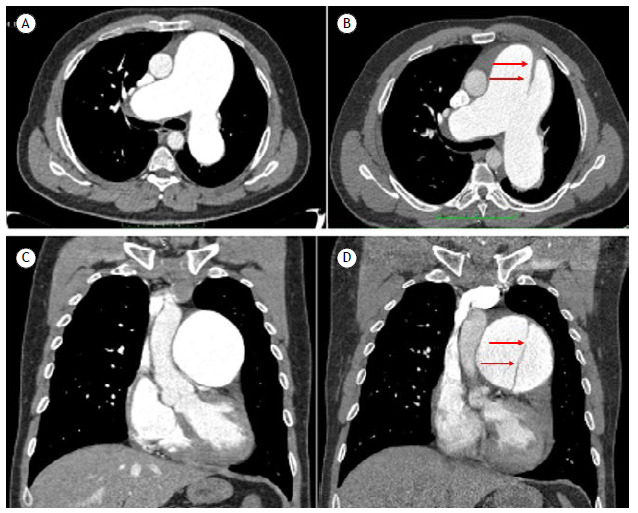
Initial chest computed tomography (CT) images showing aneurysmal dilatation of the pulmonary artery trunk and its main branches in axial (A) and coronal (B) views. On the right, follow-up CT images obtained six months later revealing a dissection flap in the pulmonary artery trunk (arrows in C and D).
